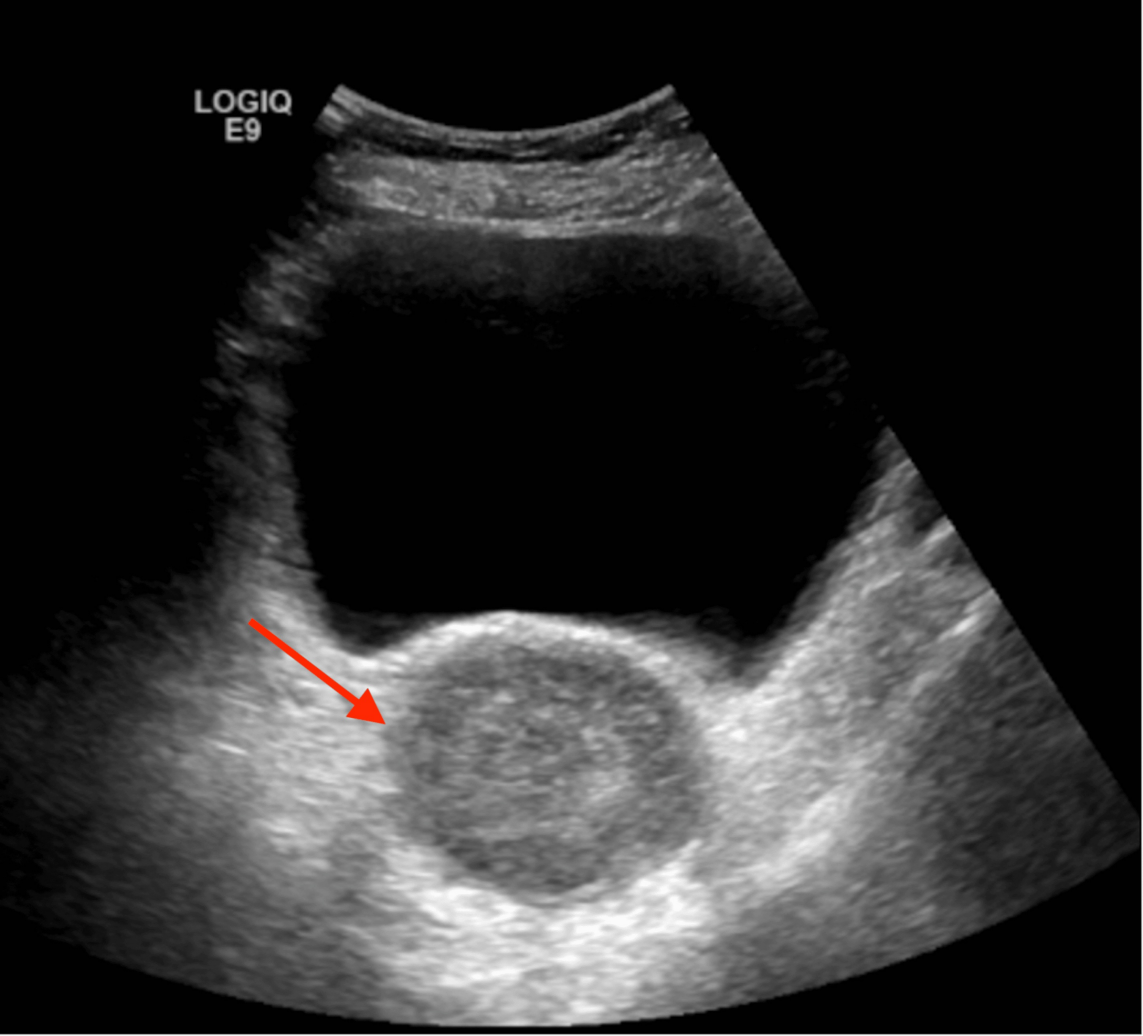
Pelvic ultrasound Infiltrative uterine mass
